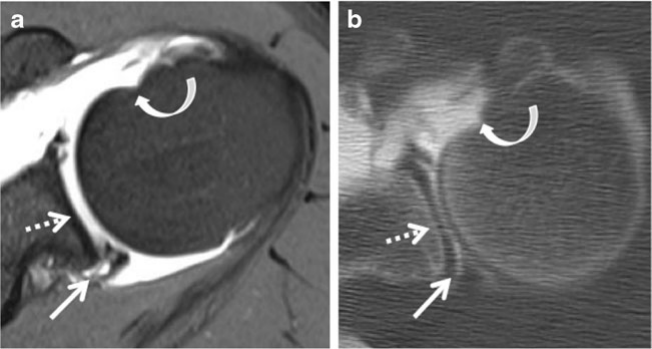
Posterior instability in a 32-year-old male athlete following a ski fall on a flexed elbow and shoulder a year earlier. Axial (a) fat-suppressed T1-weighted MR arthrography and (b) corresponding CT arthrography images. Posterior labral tear consistent with reverse Bankart tear (*straight arrow*). Cartilage thinning of the glenoid (*dashed arrow*) and anterosuperior humeral head impaction (*curved arrow*), consistent with reversed Hill-Sachs. The labral injury extends to the superior labrum (not shown)
